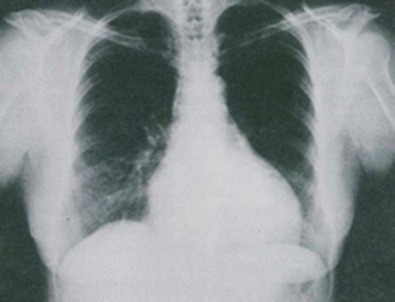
Imagen 1 Chronic chagas cardiopathy chest X-ray heart enlargement
